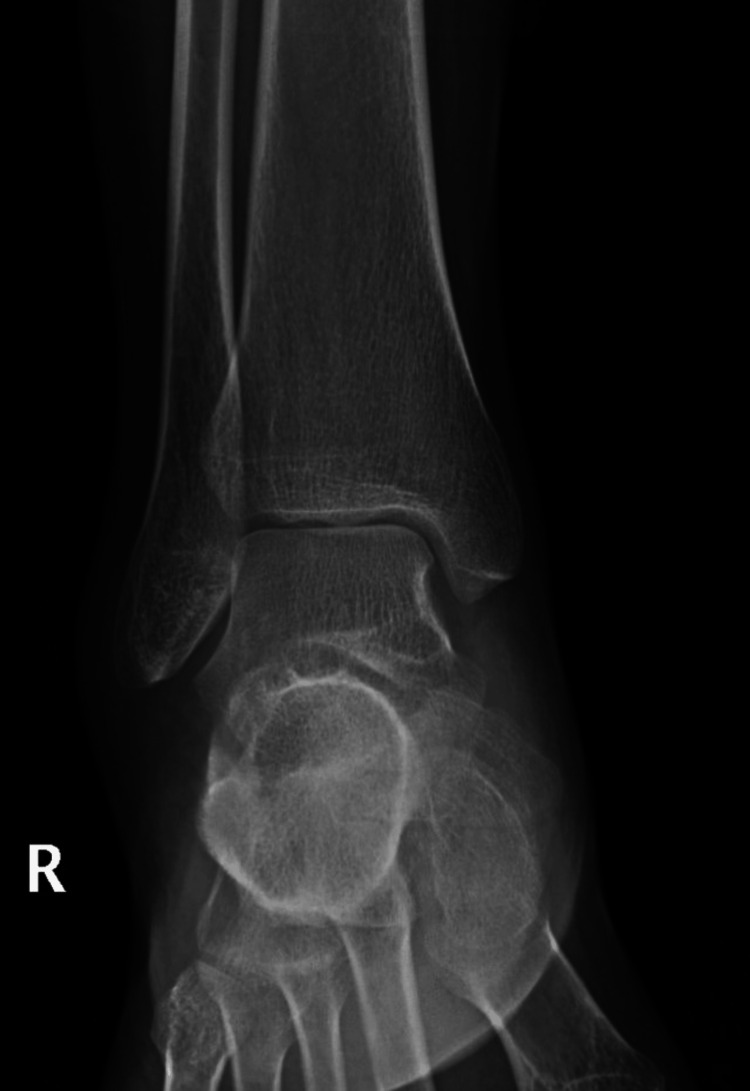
Anteroposterior view X-ray of the right ankle at the time of presentation
